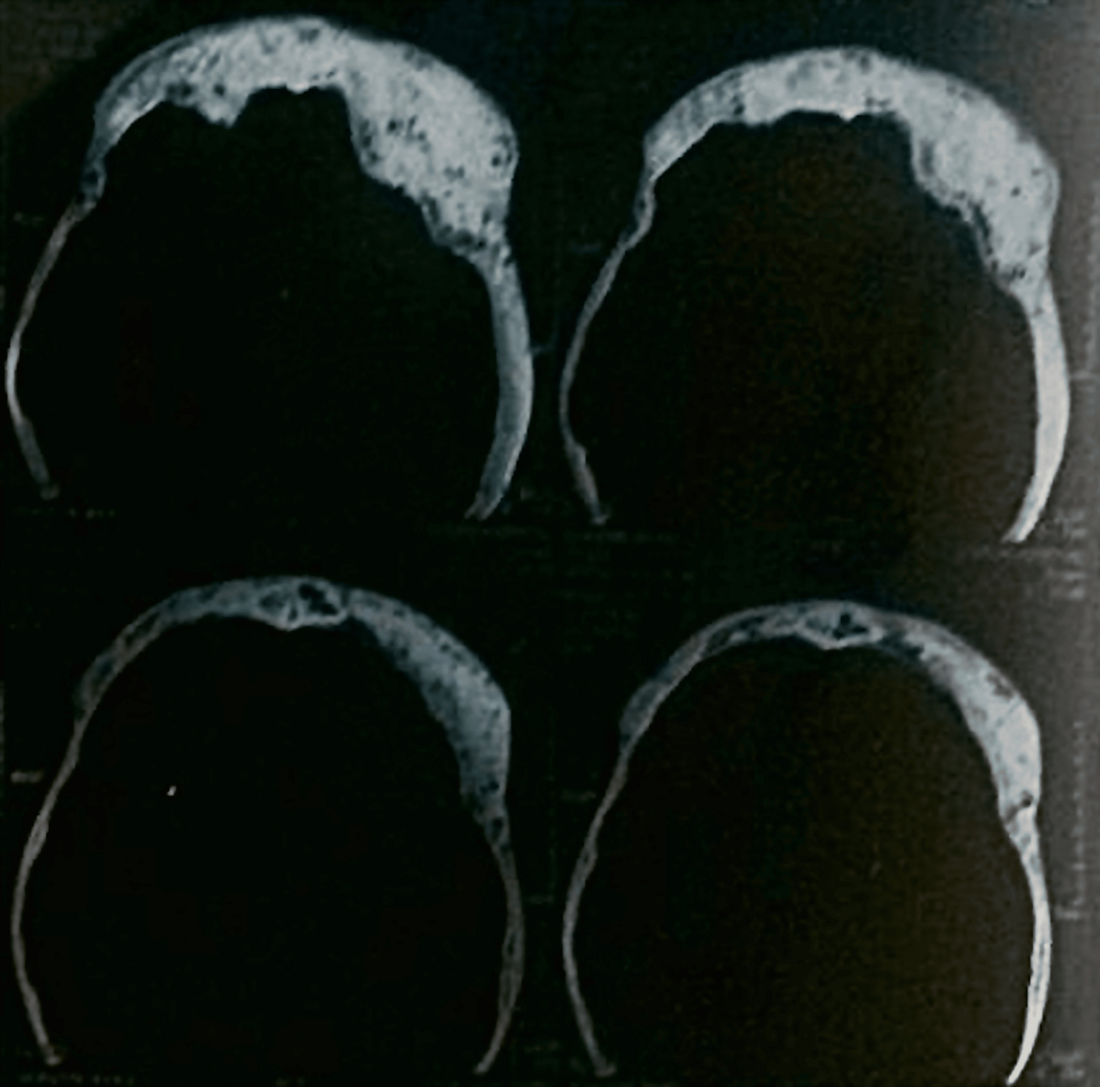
T2 MRI head post gadolinium showing cranial involvement of fibrous dysplasia
